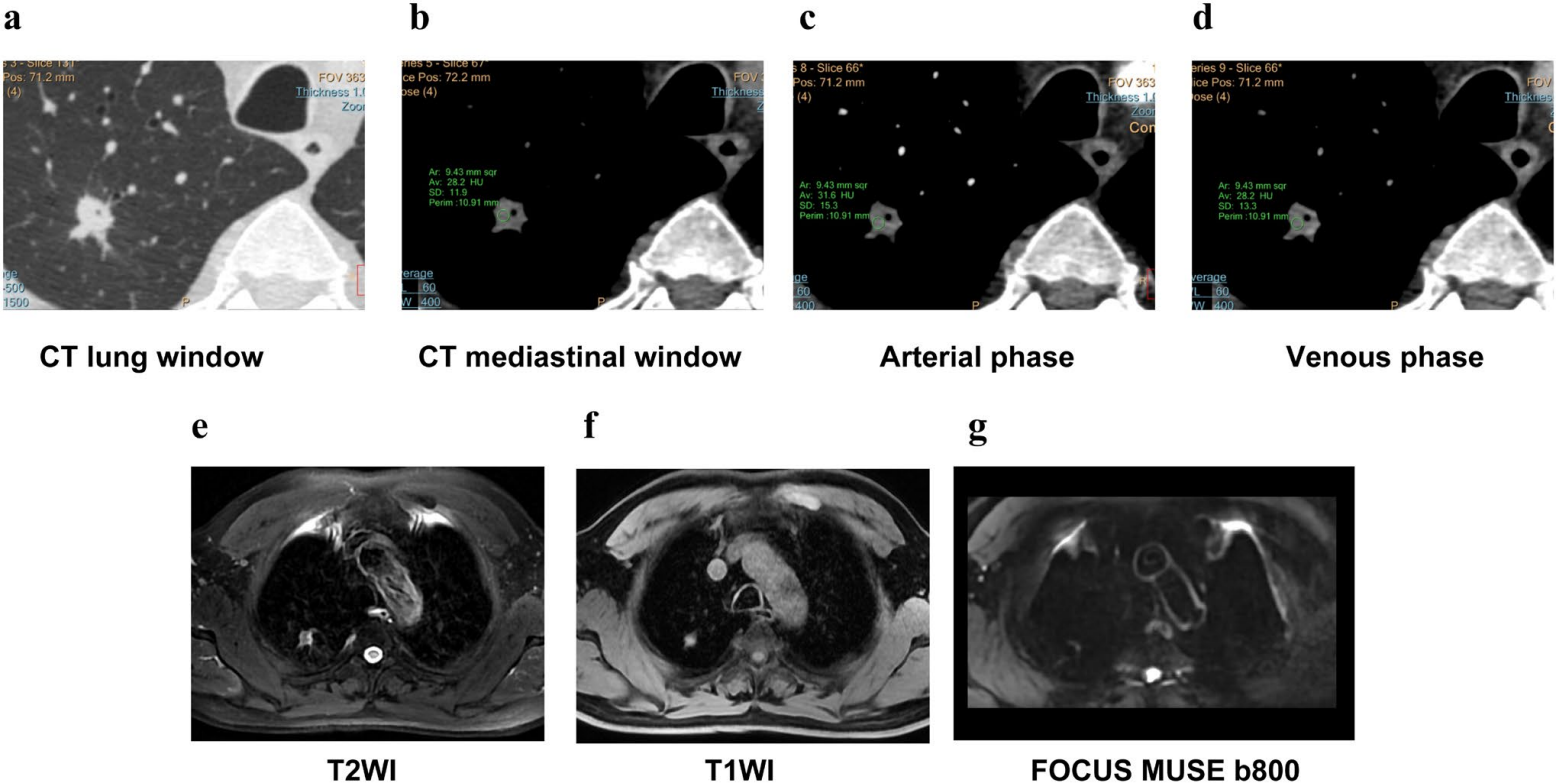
Male, 62 years old, solid nodule in the upper lobe of the right lung, approximately 24 mm in size, pathologically confirmed as tuberculosis. (a) CT lung window; (b) CT mediastinal window; (c) enhanced CT arterial phase mediastinal window; (d) enhanced CT venous phase mediastinal window; (e) T2WI; (f) T1WI; and (g) FOCUS MUSE DWI (*b* = 800 s/mm^2^). The lesion demonstrated no enhancement on contrast‐enhanced CT and exhibited low signal intensity on DWI sequences of MRI.
